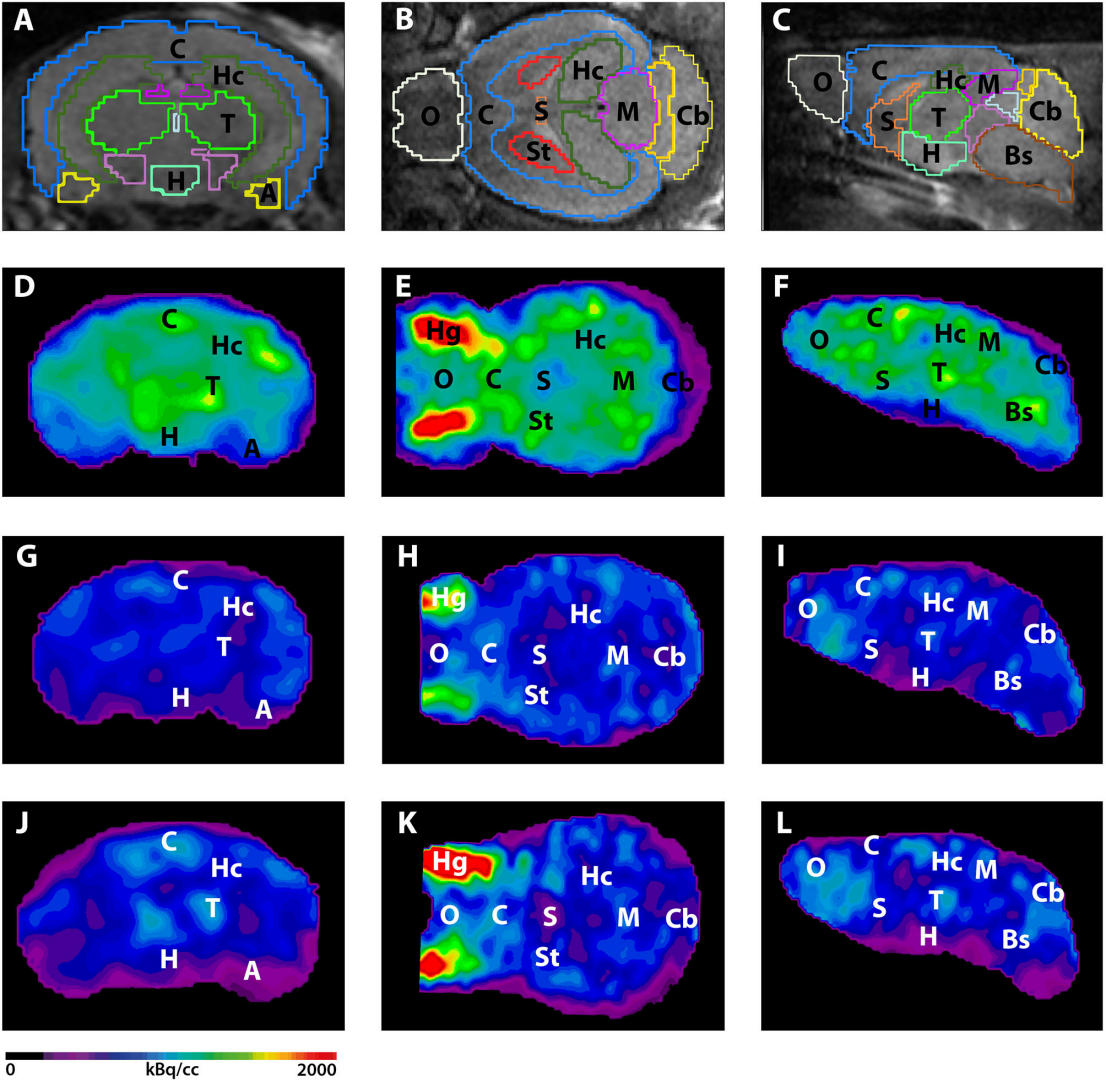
^18^F-FDG-PET images in coronal, transverse, and sagittal view. (A–C) MRI images with predefined brain regions. (D–F)
